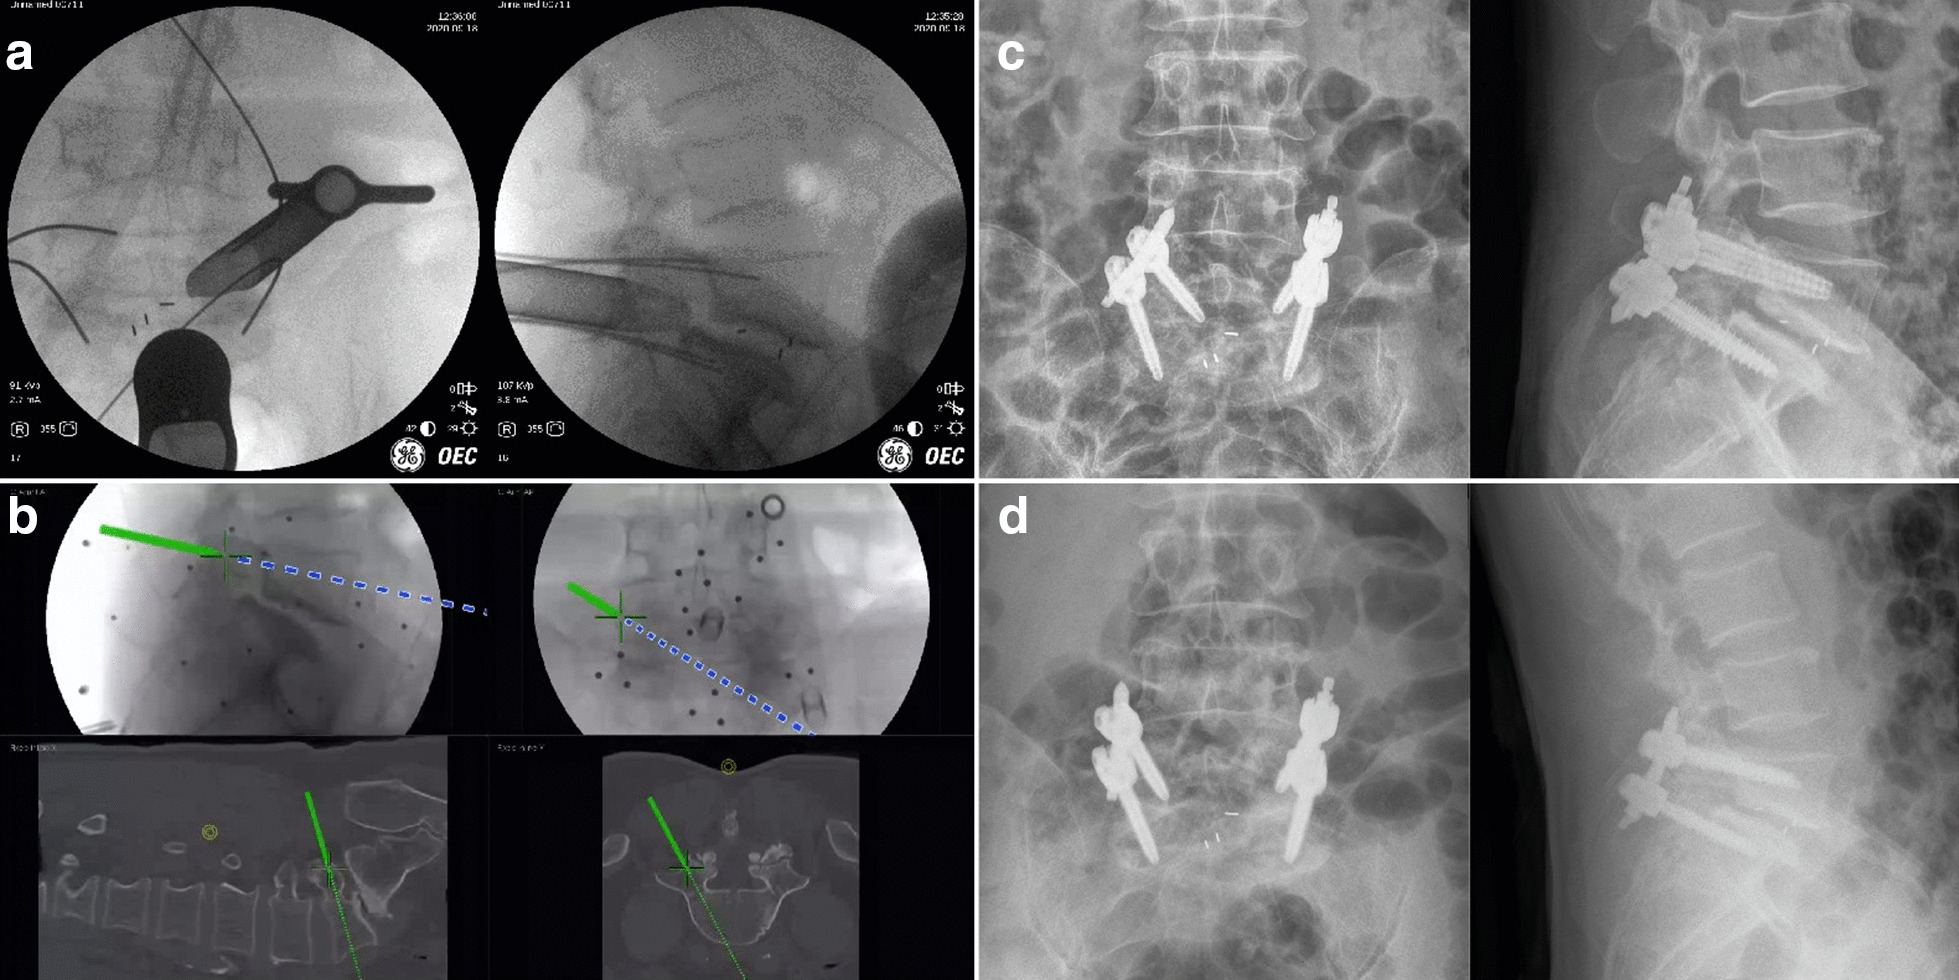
Images obtained in Case 2. a The X-ray fluoroscopy after cage insertion showed that the position of the left L5 pedicle guide wire was not satisfactory. b The surgeon adjusted the direction of the screws under the guidance of navigation again, ignoring the change in anatomical structure after slippage reset and intervertebral space restoration. c Postoperative X-ray fluoroscopy showed that the position of the left L5 pedicle screw was inward and upward, and the patient suffered from left lower extremity pain and numbness. d After a revision surgery to replace the left L5 pedicle screw, his left lower extremity symptoms were significantly relieved
